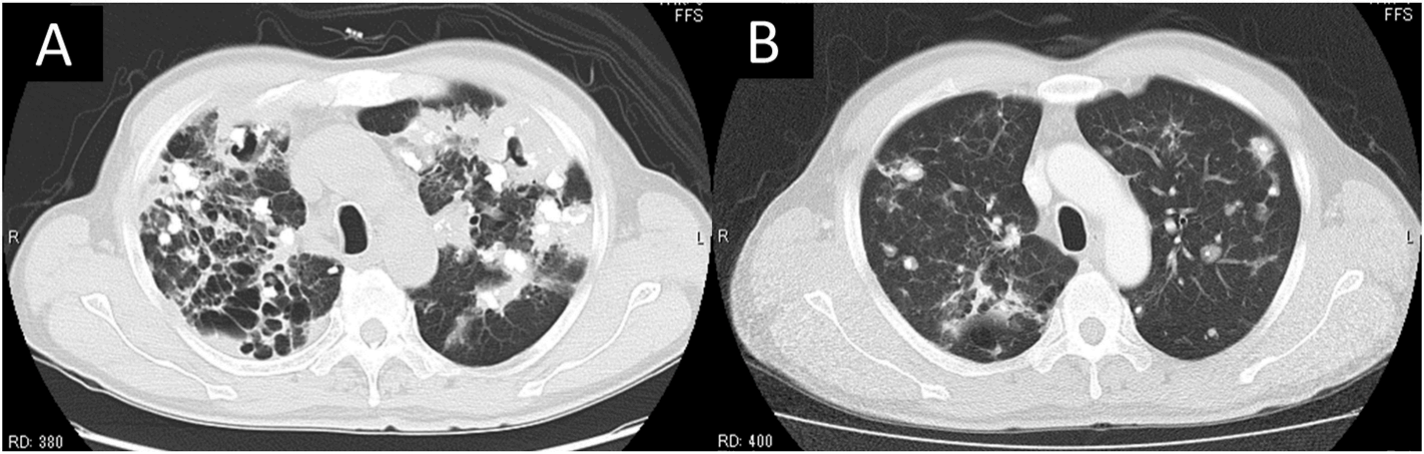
Chest CT imaging on admission showed multiple nodules and masses with calcification and cysts in both lungs (A). A CT scan of the chest 10 years earlier (B) already showed the presence of calcified nodules, which were smaller than on the present CT images.
